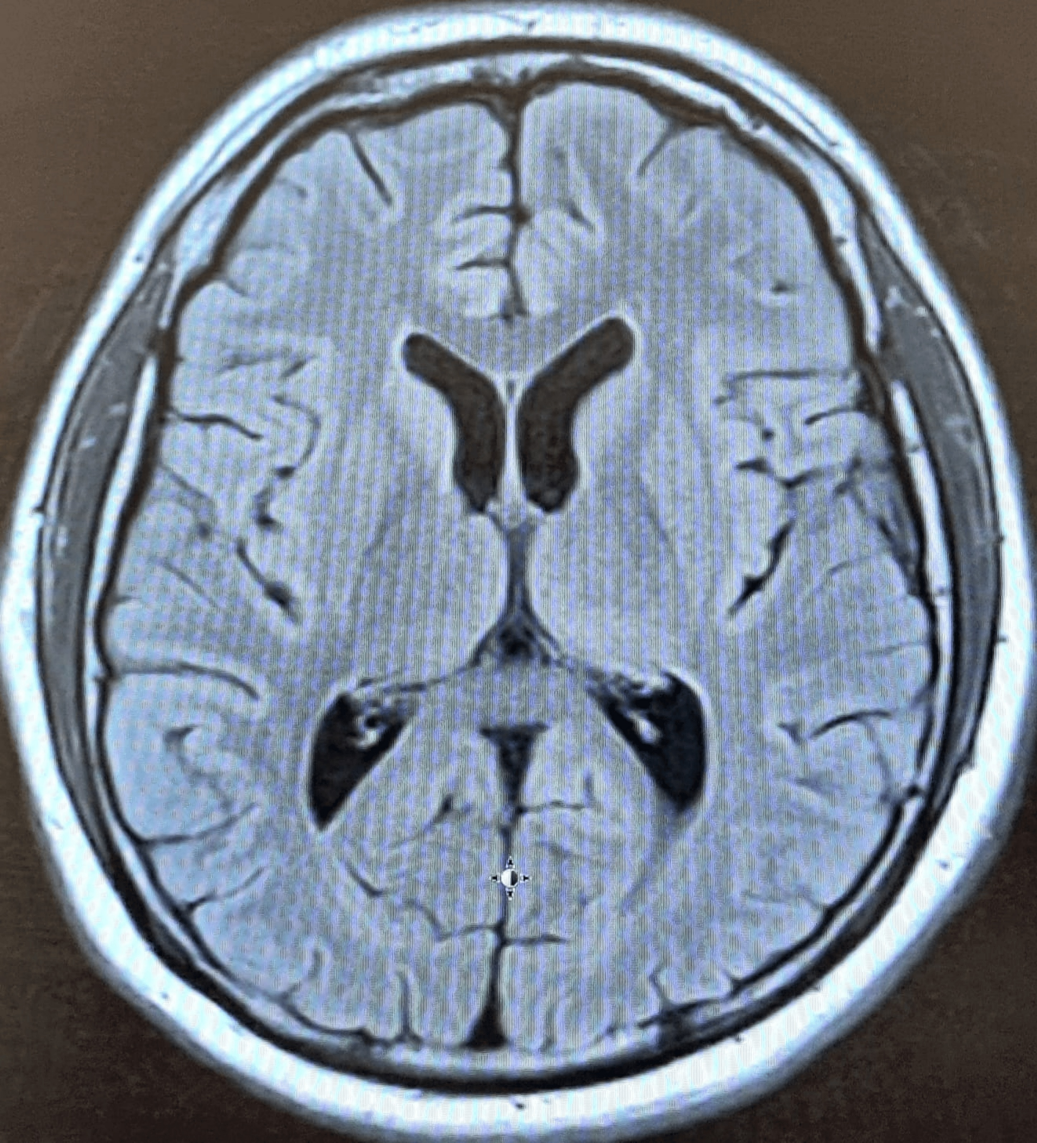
The MRI of Case 3's brain (axial FLAIR image) shows no gross pathology.
